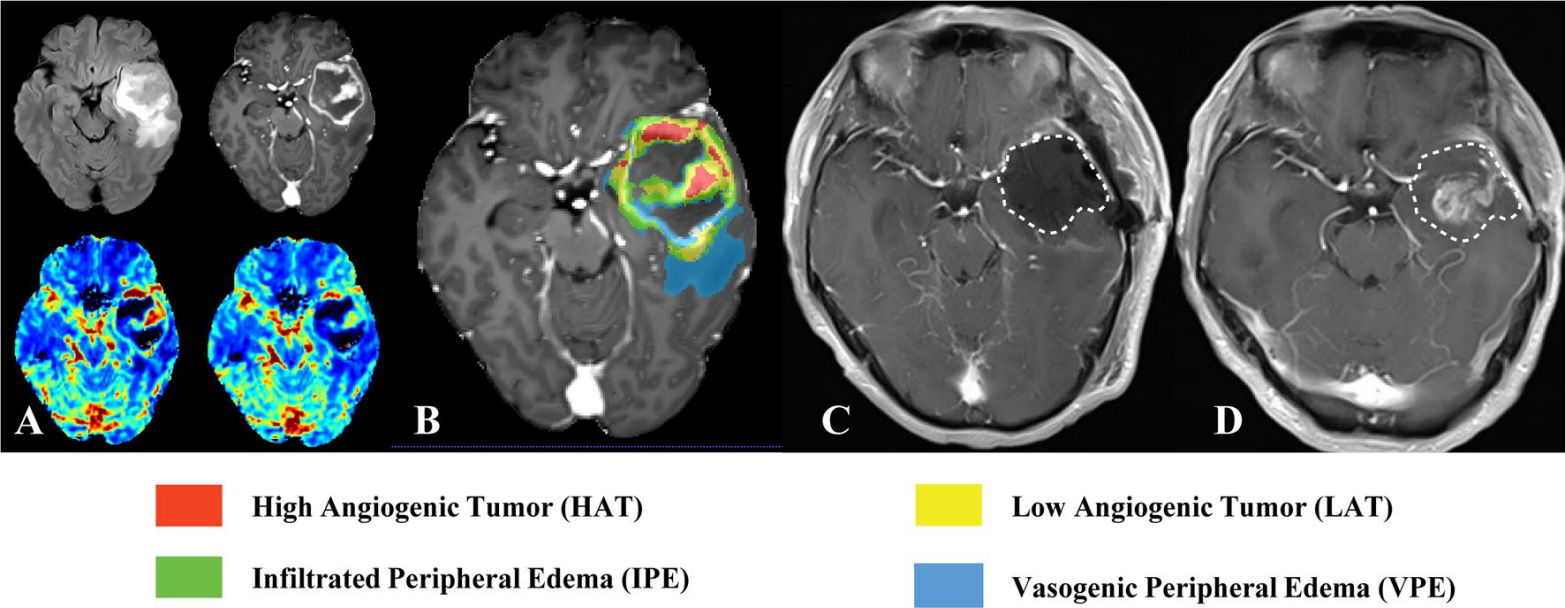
MRI scans in a 55-year-old man with glioblastoma, isocitrate dehydrogenase (IDH) wild-type, WHO grade IV, and tumor recurrence in the intra-resection cavity. Axial (A) fluid attenuation inversion recovery (FLAIR) image, contrast-enhanced T1-weighted image, cerebral blood volume (CBV) map, and cerebral blood flow (CBF) map are shown in small images (left to right, top to bottom). B Vascular habitat maps. The different colors indicate subregions of segmentation. C Twenty-four to 72 h postoperatively MRI. Contrast-enhanced T1-weighted image displayed gross total resection of the tumor and without enhancement of the surgical residual cavity (white dashed line). D Follow-up MRI. Contrast-enhanced T1-weighted image showed the tumor recurrence in the intra-resection cavity after 3 months
